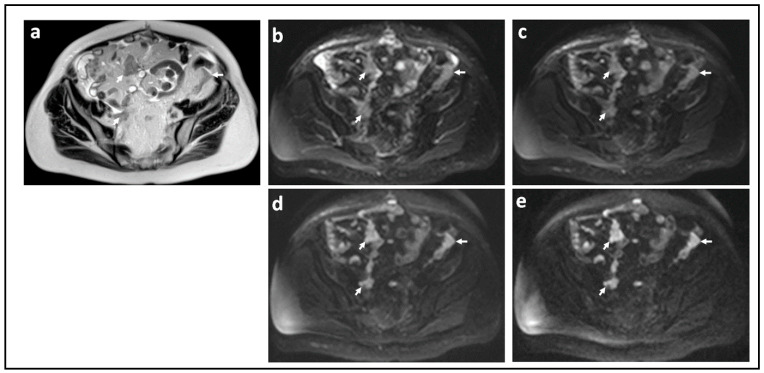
Peritoneal metastases in high grade serous ovarian cancer showing increasing lesion conspicuity with increasing *b*-value: T2-W (a) and DW-MRI at *b*-values of 0 s/mm^2^ (b), 100 s/mm^2^ (c), 500 s/mm^2^ (d), and 900 s/mm^2^ (e). The irregular metastatic deposits on the surface of the bowel and in the mesentery (arrows) show diffusion restriction. They increase in conspicuity and contrast as diffusion weighting increases because they retain signal while signal from adjacent normal tissues diffuses away.
